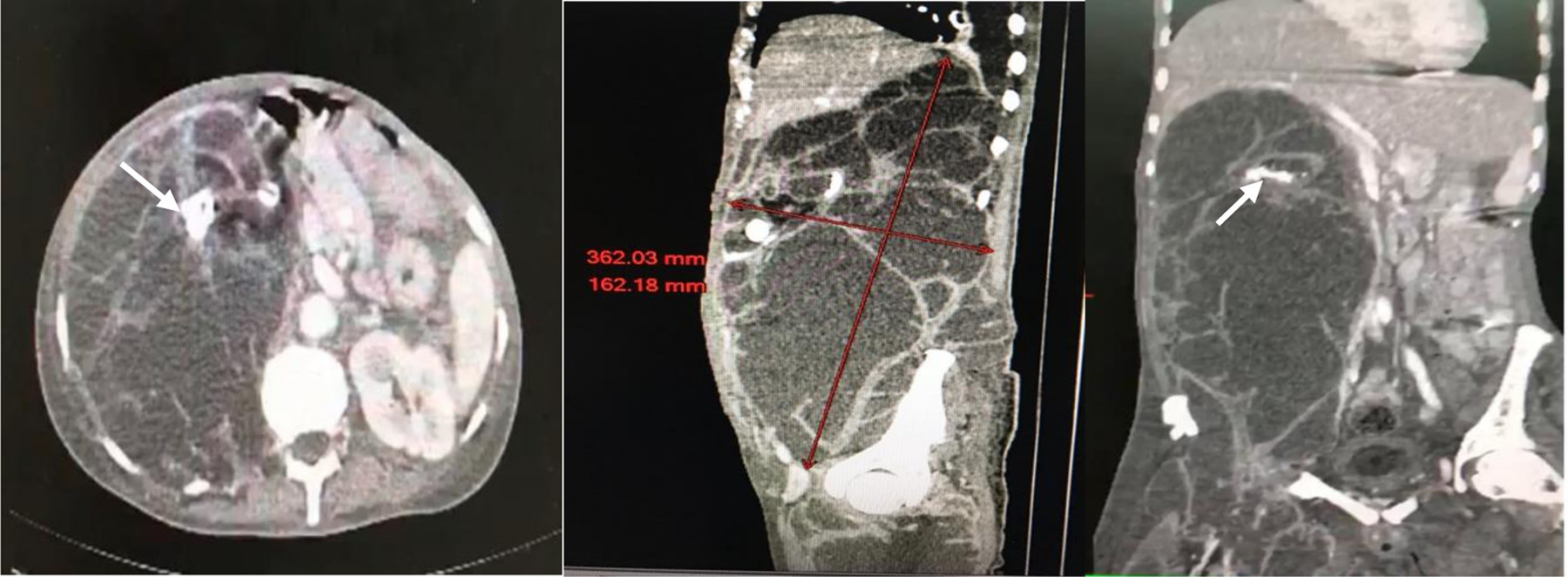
Abdominal and pelvic enhanced-contrast CT: transverse section (A), sagittal (B) and coronal (C) reconstructions showing a large collection extending from the right hypochondrium to the pelvis with enhanced septa and stones (withe arrow) without any visible renal parenchyma.
